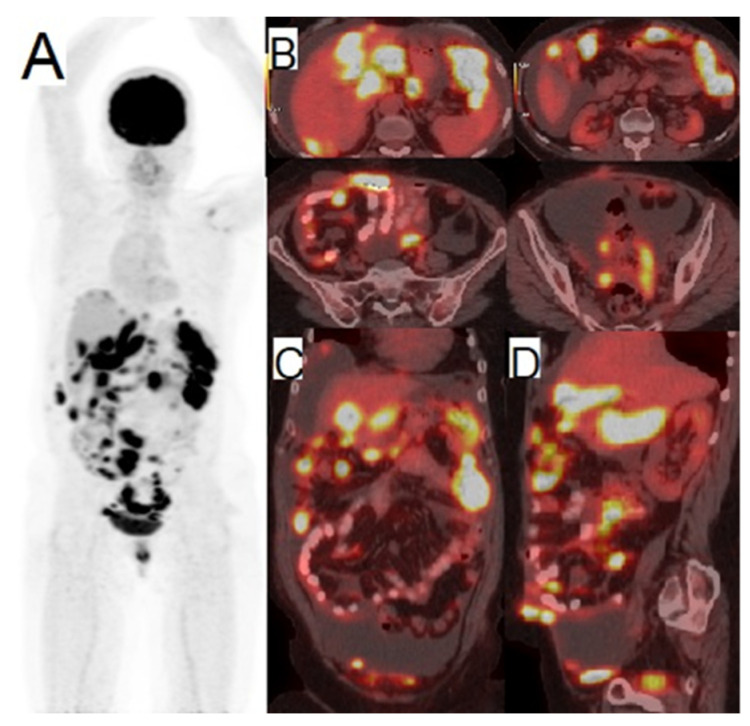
A 50-year-old female with a history of ovarian cancer, seven months post total abdominal hysterectomy with bilateral salpingo-oophorectomy (THA-BSO) with complaints of abdominal pain end measures of elevated tumor markers. (A) Anterior maximal intensity projection (MIP) [^18^F]FDG PET/CT image. (B) transvers, (C) coronal and (D) Sagittal abdominopelvic fused PET/CT images showed disseminated intraperitoneal hypermetabolic tumor seeding in abdomen and pelvic cavity as well as hypermetabolic omental thickening (omental cake) and ascites. (Image with courtesy of the Department of Nuclear Medicine, Vali-Asr Hospital, Tehran University of Medical Sciences, Tehran, Iran).
